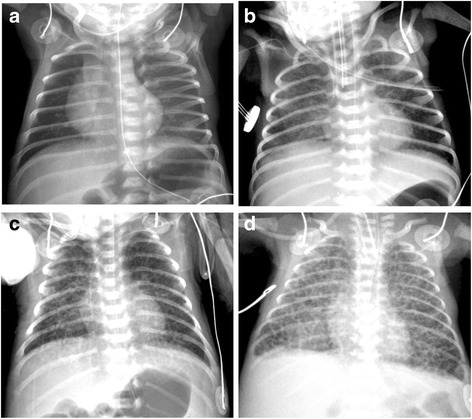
Chest radiographs representing each grade. a No demonstrable abnormality (grade 1). b Granular infiltration (grade 2). c Diffuse streaky interstitial thickening (grade 3). d Diffuse coarse interstitial thickening (grade 4)
